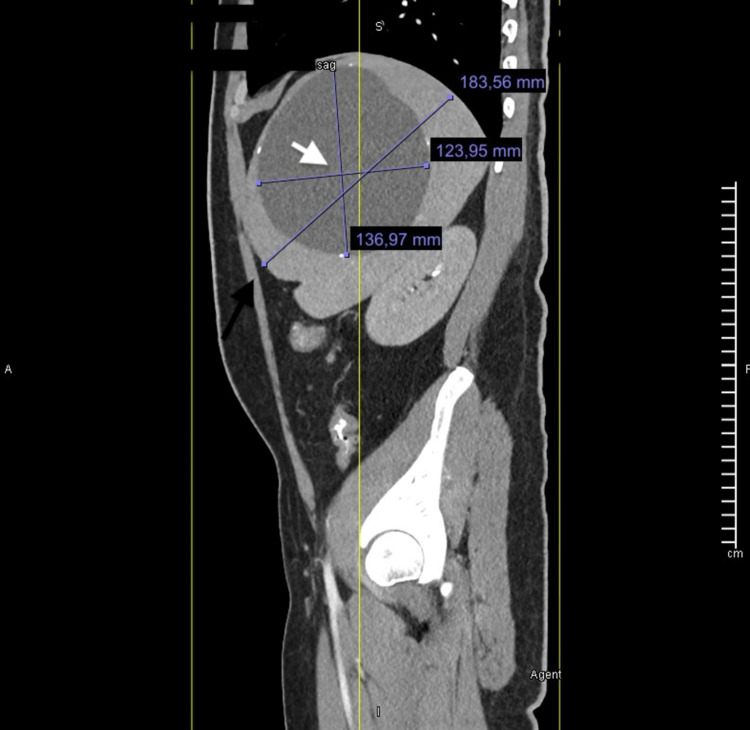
Contrast-enhanced computed tomography (sagittal view). Splenic measurement of 183.56 mm (black arrow). Splenic cyst measurement of 123.95 × 136.97 mm (white arrow).
